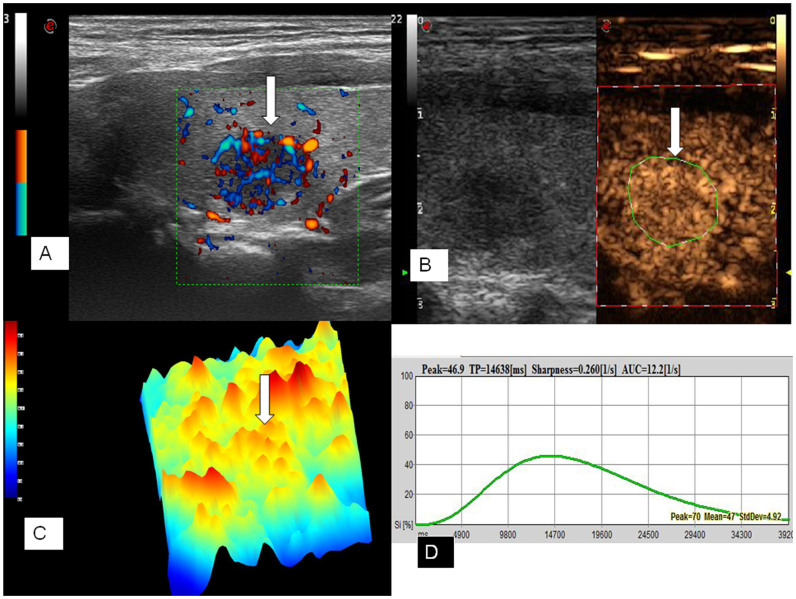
Ultrasound images of a 26-year-old female PTMC patient with CCLNM of the left thyroid lobe. Power Doppler image showed rich blood signals (arrow) in the tumor (A). CEUS image showed diffuse and homogeneous enhancement (arrow) across the whole lesion (B). Parametric map showed that the nodule was in yellow and red (arrow), which indicates hyperenhancement with respect to adjacent thyroid parenchyma (in blue) (C). Numeric values of peak, TP, sharpness, and AUC were automatically calculated based on the time–intensity curve and demonstrated on the top of form (D).
